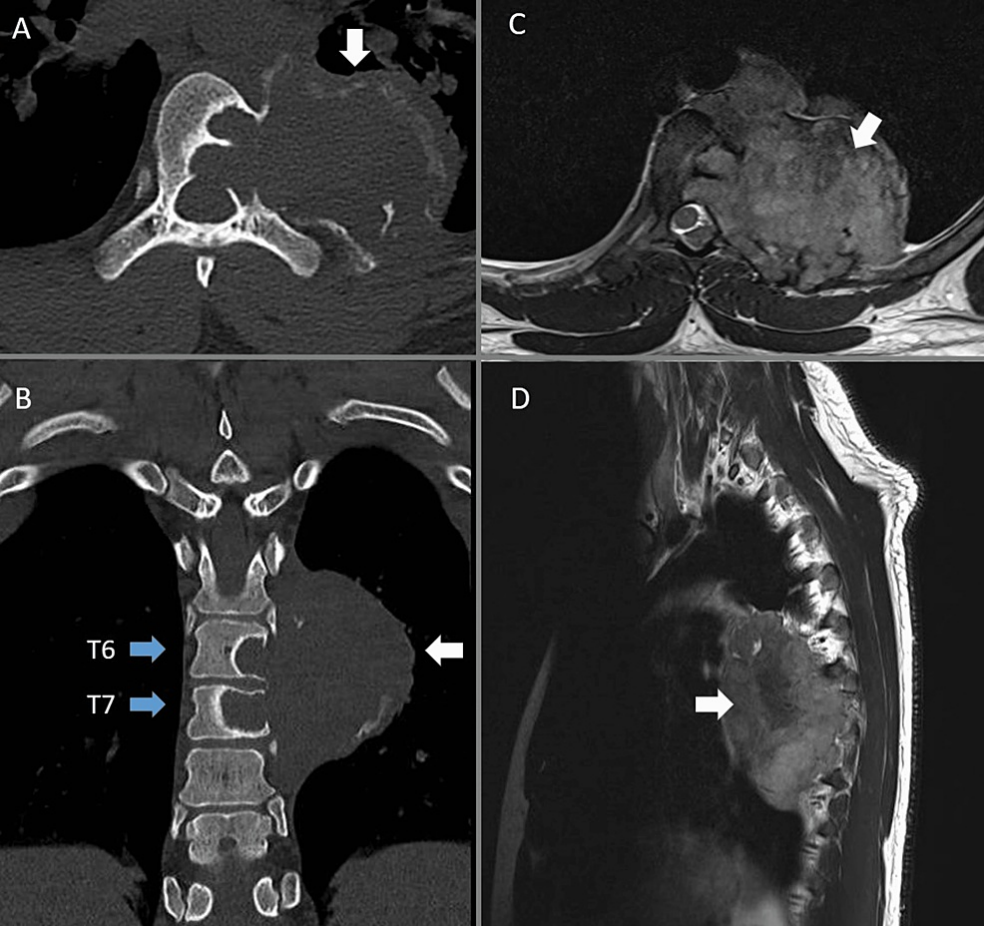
Case One Twenty-seven-year-old male with a giant cell tumor. A and B demonstrate axial and coronal CT views with lytic tumor evident within T6 and T7 (blue arrows), the extraosseous extend from T5-T8 can be seen in A and B (white arrows). C and D show axial and sagittal T2 MRI views with evident tumor mass (arrows).
